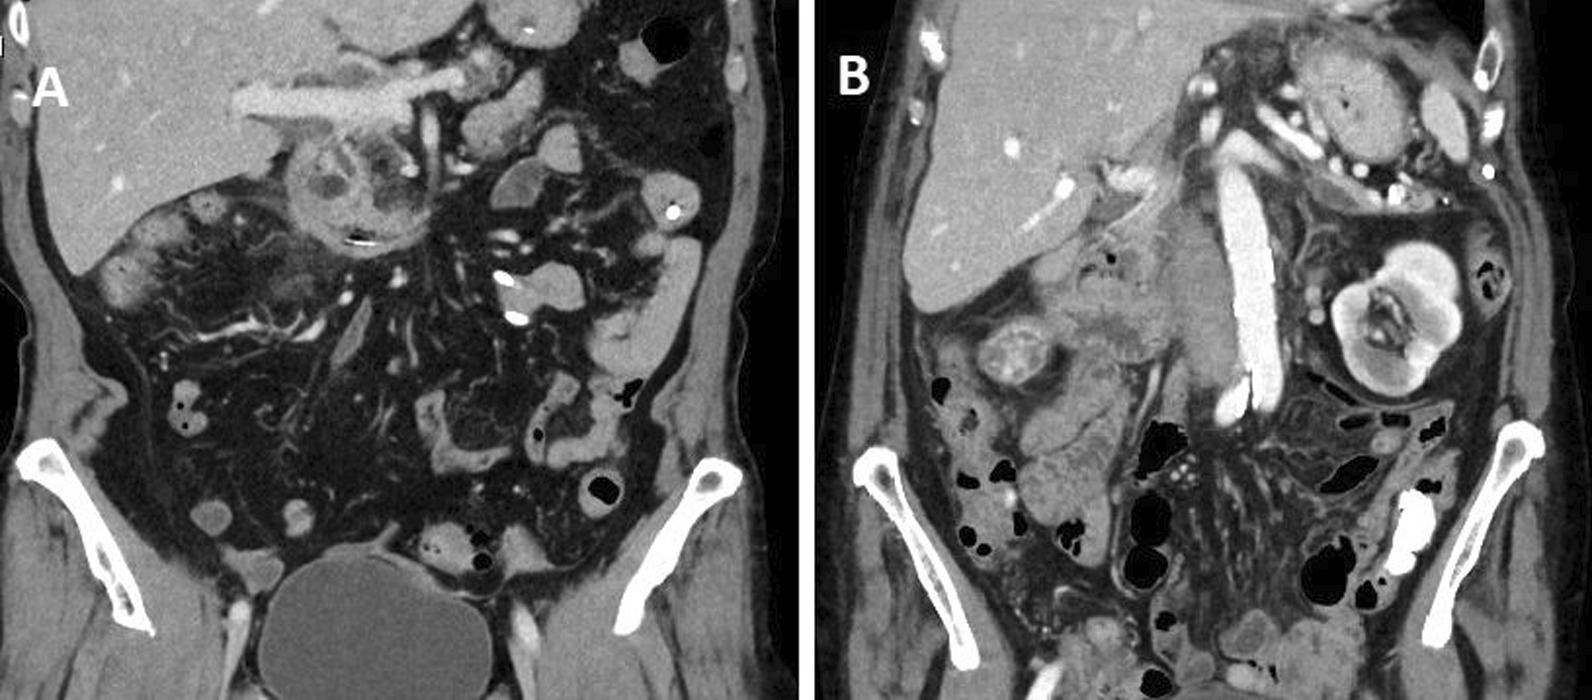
Last CT-scan prior discharge of Patient #1 (a) and Patient #2 (b)
